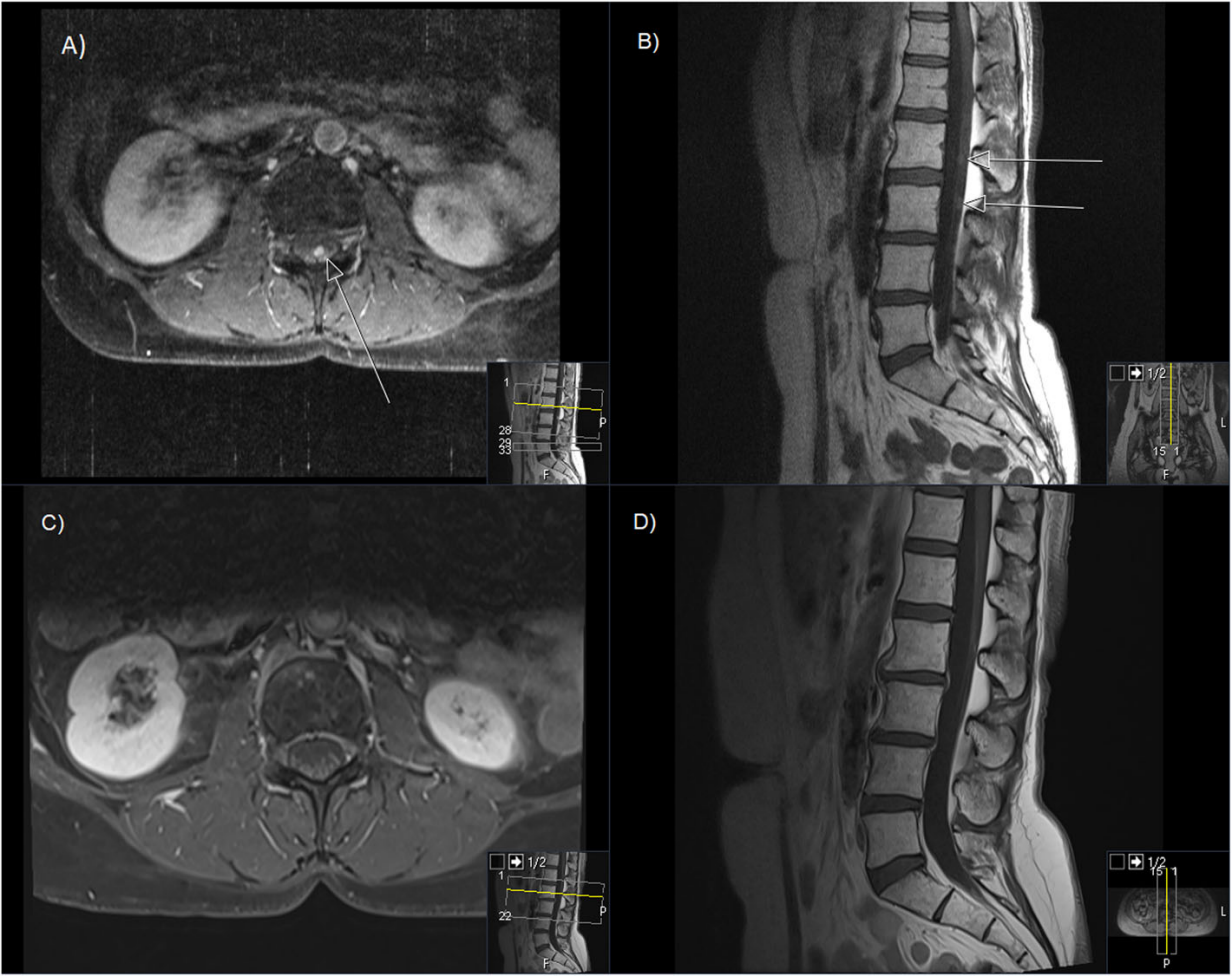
Example of a patients’ MRI before and 6 months after CSI. Contrast enhanced T1 MRI sequence A,B) Prior to CSI, C,D) 6- months post CSI; A,C) axial view B,D) sagittal view. Arrows point at macroscopic intraspinal disease
